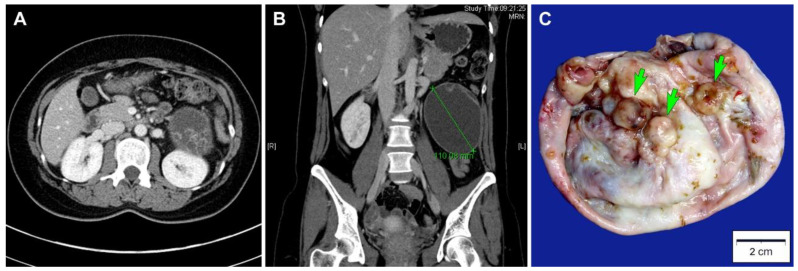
Imaging and gross findings. (A) Abdominopelvic computed tomography scan in axial view, which revealed a thick-walled cystic mass in the left retroperitoneal space. The mass had some daughter cysts. (B) Abdominopelvic computed tomography scan in coronal view, which revealed a well-circumscribed, ovoid unilocular cystic mass with a diameter of 11 cm. (C) Grossly, the inner surface of the mass showed some round-to-ovoid, variegated mural nodules (green arrows), measuring up to 1.2 cm in the greatest dimension.
